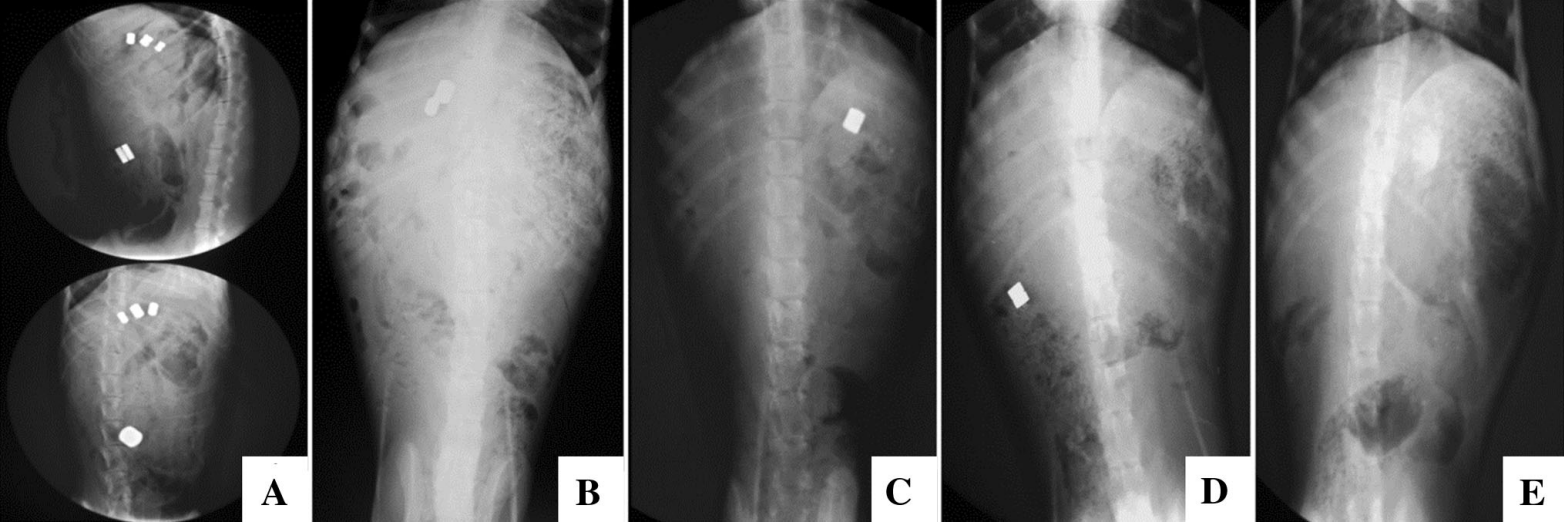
The expelling process of MCAs was monitored by X-ray. A The instant fluoroscopy showed all anastomats coupled well and retained at the right place (*upper* lateral view; *lower* anteroposterior view). B At 4th day after RYHJ, EE-MCA and the first BE-MCA were expelled out of the body, and two BE-MCAs remained in situ. C At 8th day after RYHJ, the second BE-MCA was missing, and the third one already dropped in small intestine. D At 9th day after RYHJ, the third BE-MCA was expelled at hepatic flexure of ascending colon. E At 10th day, all anastomats were expelled out of the body
